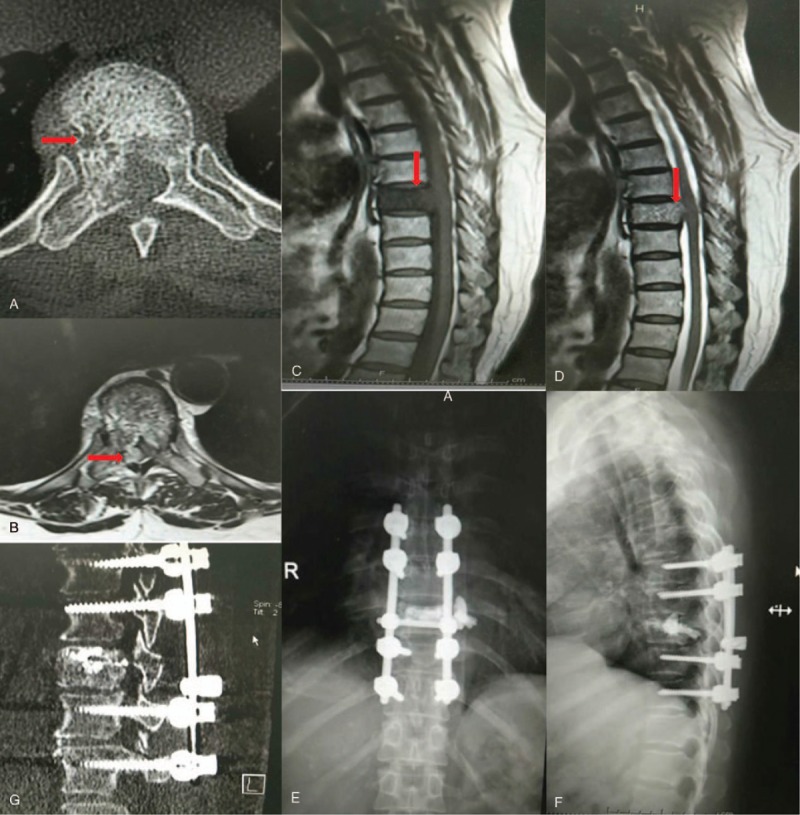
A 49-year-old female patient was diagnosed as having T9 whole vertebral hemangioma. (A) Axial computed tomography (CT) scan shows the “polka-dot” pattern of the hemangioma in T9 vertebral body (red arrow). (B) Axial magnetic resonance imaging shows ballooning of the posterior vertebral body wall and tumor, compressing the thecal sac (red arrow). (C, D) Low signal and high signal of the T9 vertebral body is seen in the T1 and T2 weighted image (red arrow), respectively. (E, F) X-ray 12 months after operation indicates that the height of T9 vertebral body was maintained and the location of pedicle screw is perfect. (G) Sagittal CT scan shows T9 vertebral body is filled with polymethyl methacrylate.
